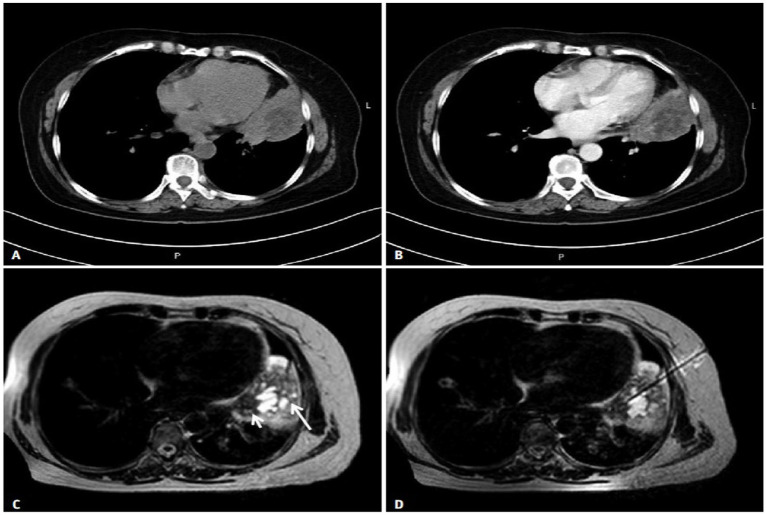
MRI-guided lung puncture. (A,B) Non-contrast-enhanced and contrast-enhanced CT did not allow reliable differentiation of tumors from the adjacent atelectasis. (C) Fast T2-weighted imaging-turbo spin echo morphological MRI performed at the same level shows post-obstructive atelectasis (long arrow) with a higher signal intensity than the central tumor (short arrow), reflecting a higher water content. (D) MRI-guided puncture needle in the lesion.
